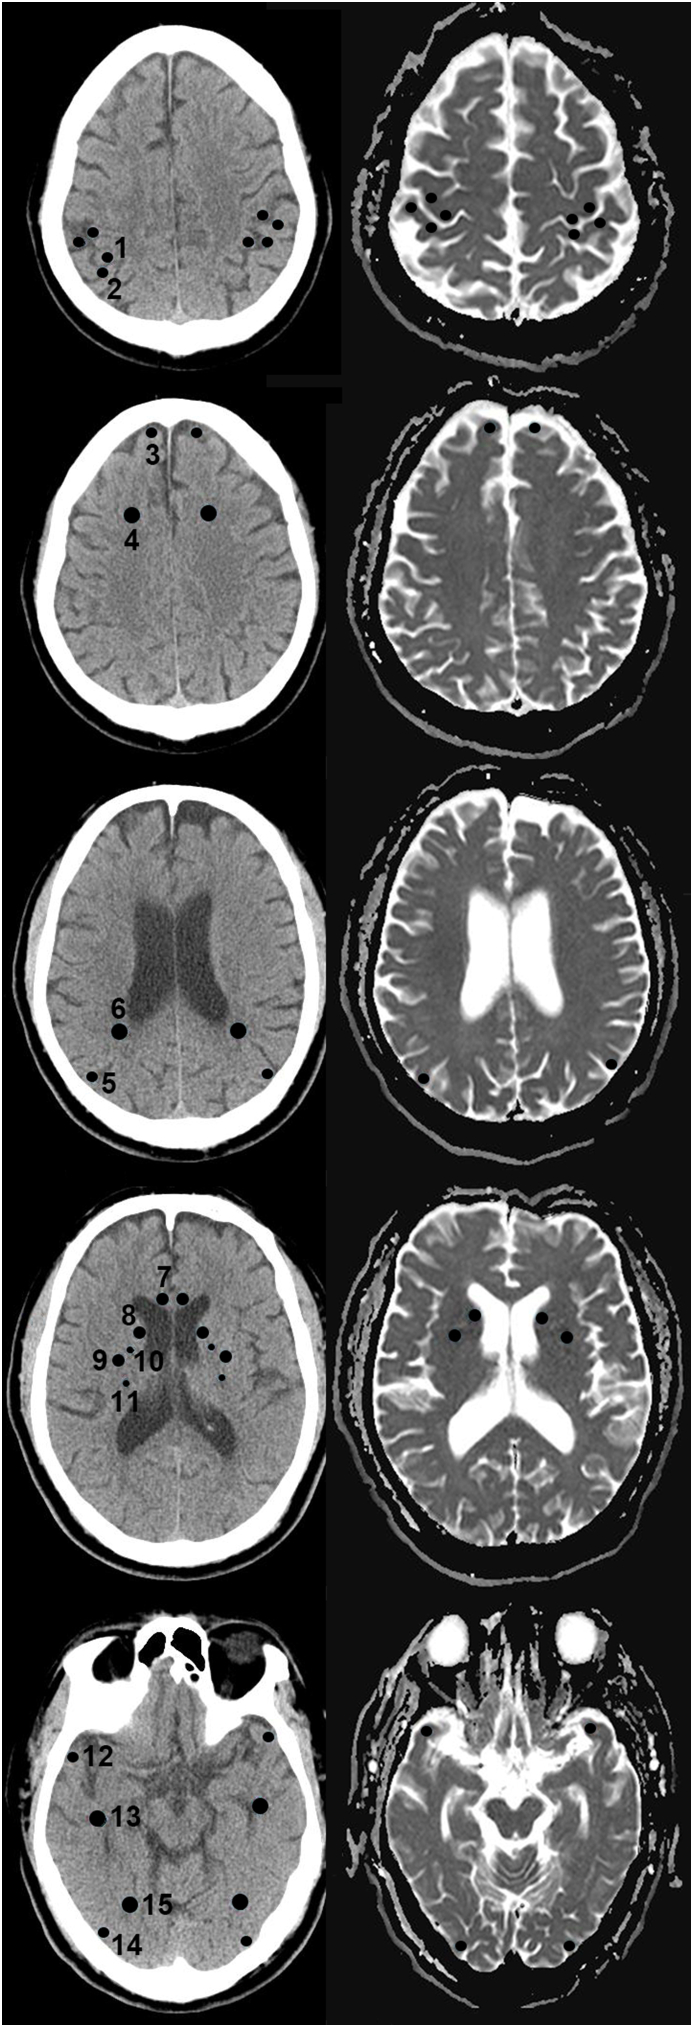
ROI regions in brain CT and MRI. (1) Precentral cortex, (2) postcentral cortex, (3) frontal cortex, (4) frontal white matter, (5) parietal cortex, (6) parietal white matter, (7) corpus callosum, (8) caudate nucleus, (9) putamen, (10) anterior limb of internal capsule, (11) posterior limb of internal capsule, (12) temporal cortex, (13) temporal white matter, (14) occipital cortex, and (15) occipital white matter.
